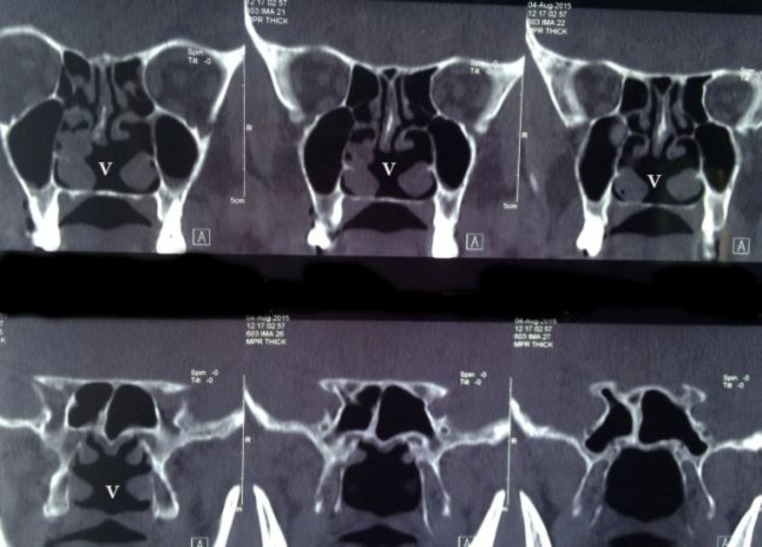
CT Scan of case 1 that shows a defect in posteroinferior part of septum. This part is in accordance to vomerian part of nasal septum (V
